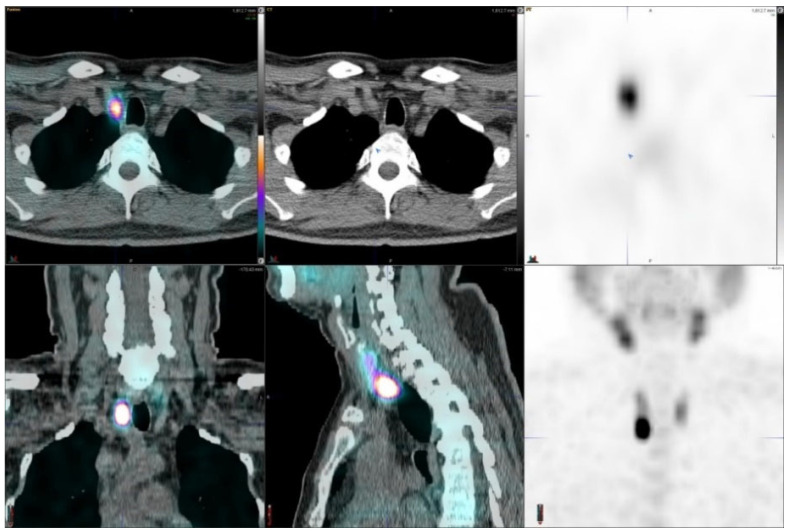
A 55-year-old male with hyperparathyroidism, right inferior parathyroid adenoma, it weighed 2.4 g. His S-PTH concentration was 162 ng/L and S-Ca 10.9 µg/L. The SUVmax values were 21.8 (CGZAS) and 16.7 (CGAS), respectively. Visually, this was a high uptake (TBR > 8) and the lesion diameter on CT was 18 mm.
